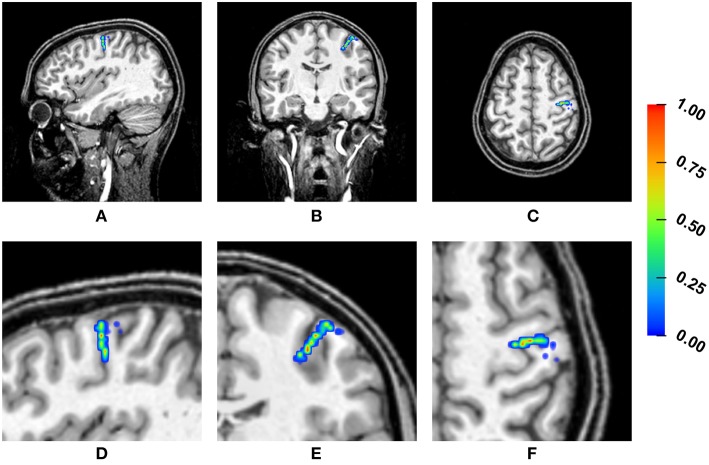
Visualization of the source localizations for the multivariate distribution overlaid on the T1-MRI showing the full head (A–C) and a detail around the source locations (D–F). From left to right showing sagittal (A,D), coronal (B,E), and axial slices (C,F). Color bar ranges from low frequency of source localizations (blue) to high frequency of source localizations (red). Values are normalized to the maximum for each slice.
